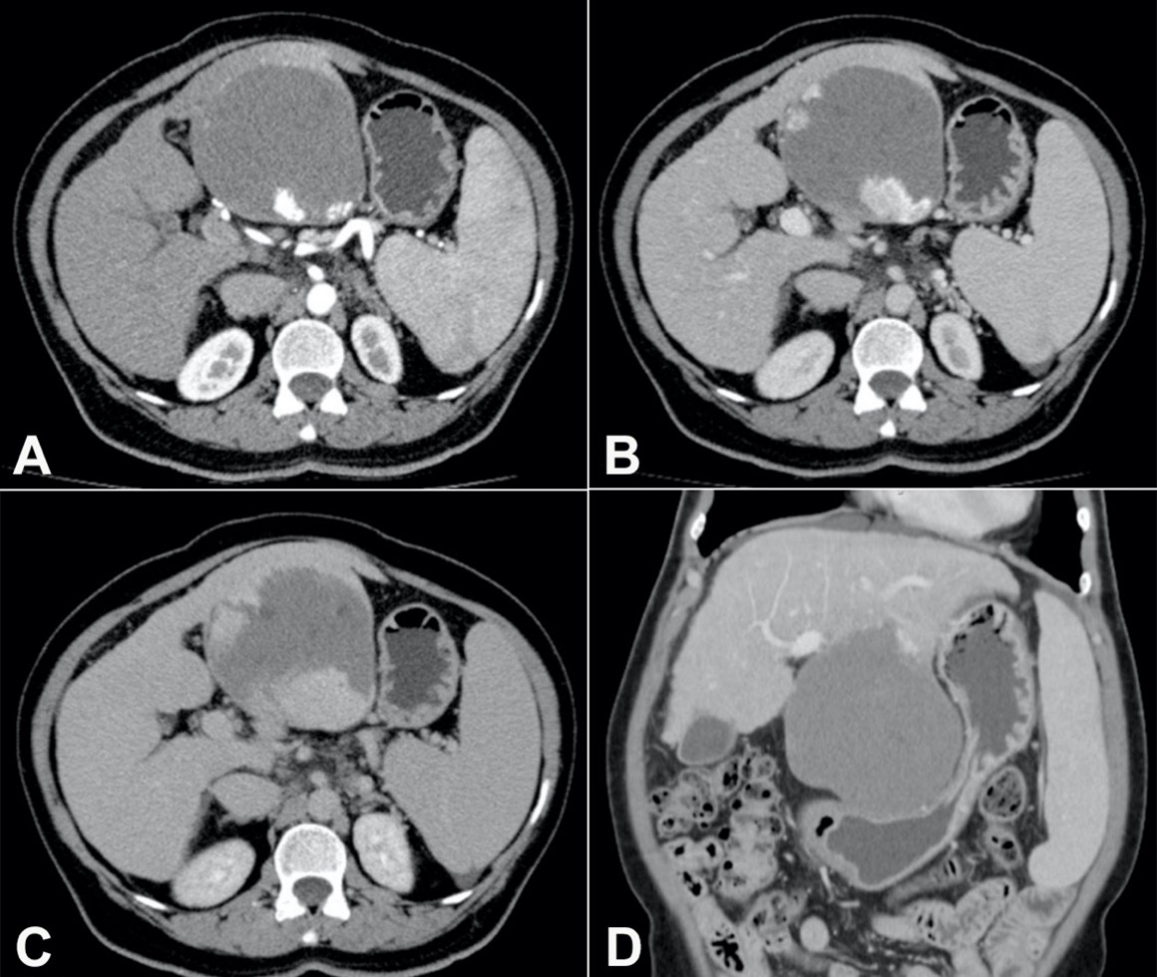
Contrast-enhanced abdominal computed tomography: axial section, in the (A) arterial, (B) venous, and (C) late phases: exophytic tumor in the left hepatic lobe (segments II and III), measuring up to 11.5 cm, with centripetal contrast enhancement, compatible with hepatic hemangioma; (D) coronal section, in the venous phase: hepatic hemangioma with significant compression of the stomach; liver with rounded borders and irregular surface, splenomegaly.
